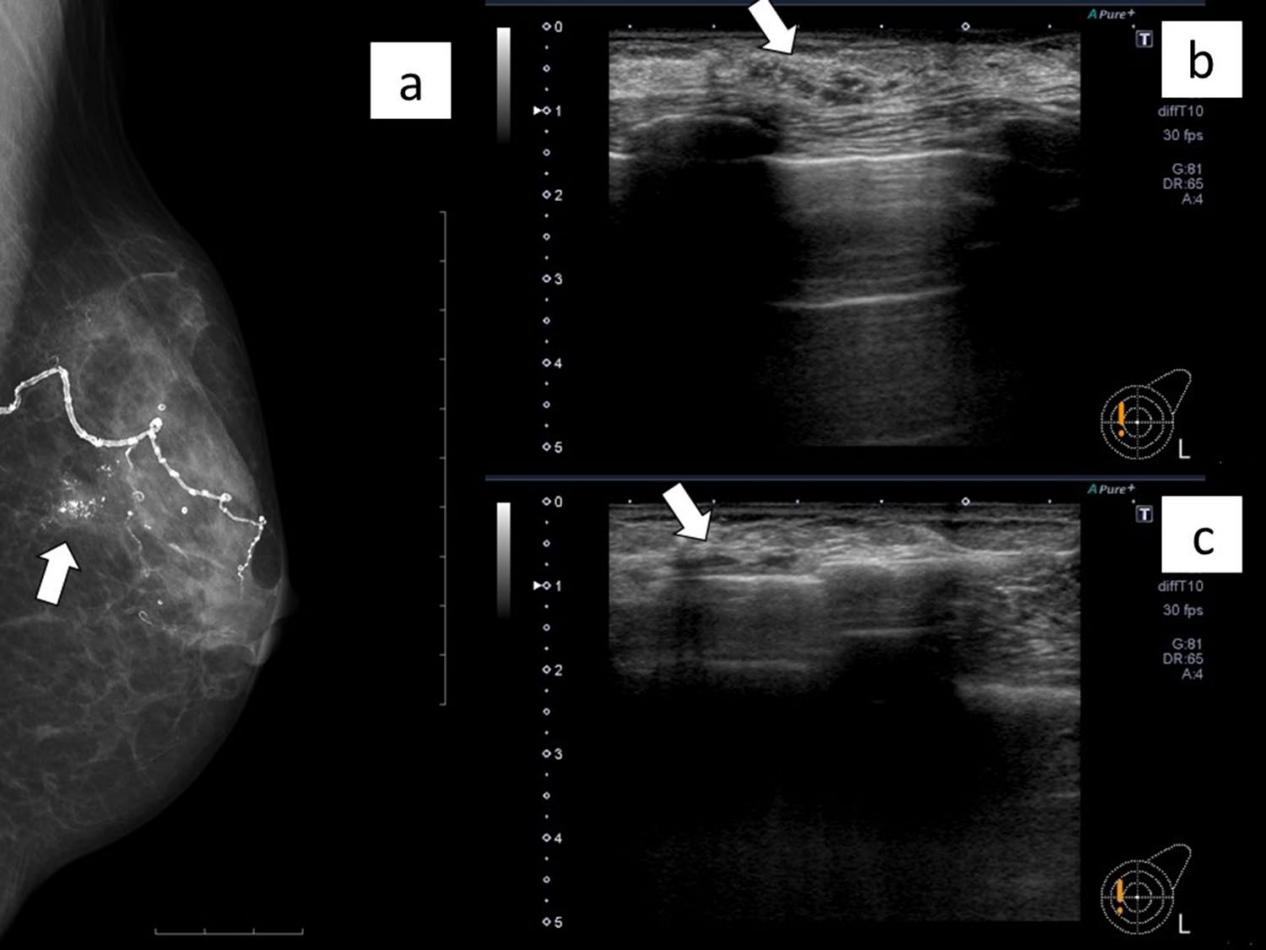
Non-mass lesion (ductal carcinoma in situ) diagnosed using vacuum-assisted biopsy. A woman in her 80s with calcified lesions on screening mammography. Mammogram showing clusters of pleomorphic calcifications in the left breast (arrow) (a). Ultrasonogram showing a 20-mm hypoechoic area with a punctate hypoechoic area corresponding to the calcifications, and the lesion was diagnosed as BI-RADS category 4 (arrow) (b). A 12-gauge spring-loaded vacuum-assisted biopsy was performed (arrow), and the lesion was diagnosed as ductal carcinoma in situ (c)
